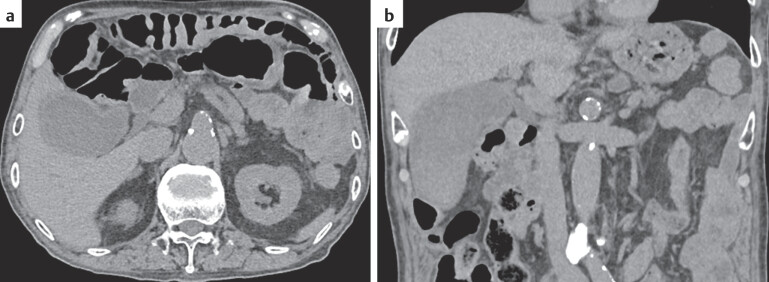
Pre-procedure computed tomography demonstrating acute
